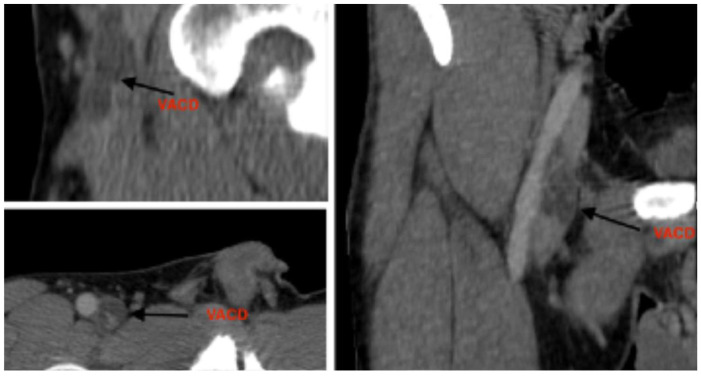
CT angiography image demonstrating venous adventitial cystic disease; the arrow indicates the cystic irregular lesion. The structure fills almost the entire lumen.
